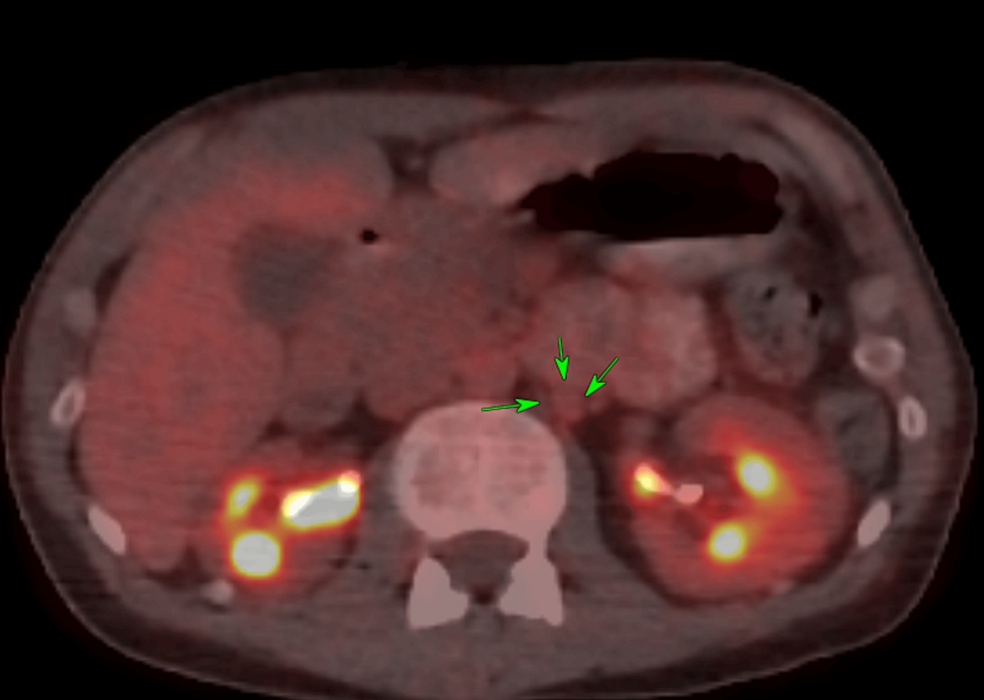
Whole-body PET-CT scan showing minimal activity in the left para-aortic lymph nodes (reactive), with no convincing evidence of disease metastasis.
